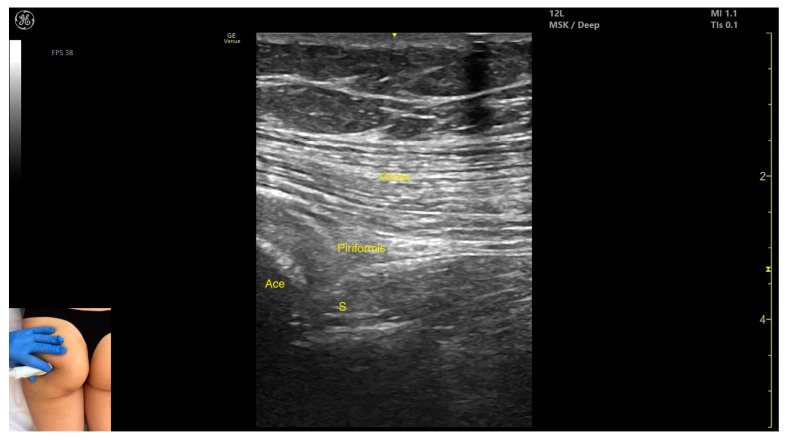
Ultrasound anatomy of *piriformis* with key landmarks: Gmax—*gluteus maximus*; Ace—acetabulum; S—sciatic nerve.
